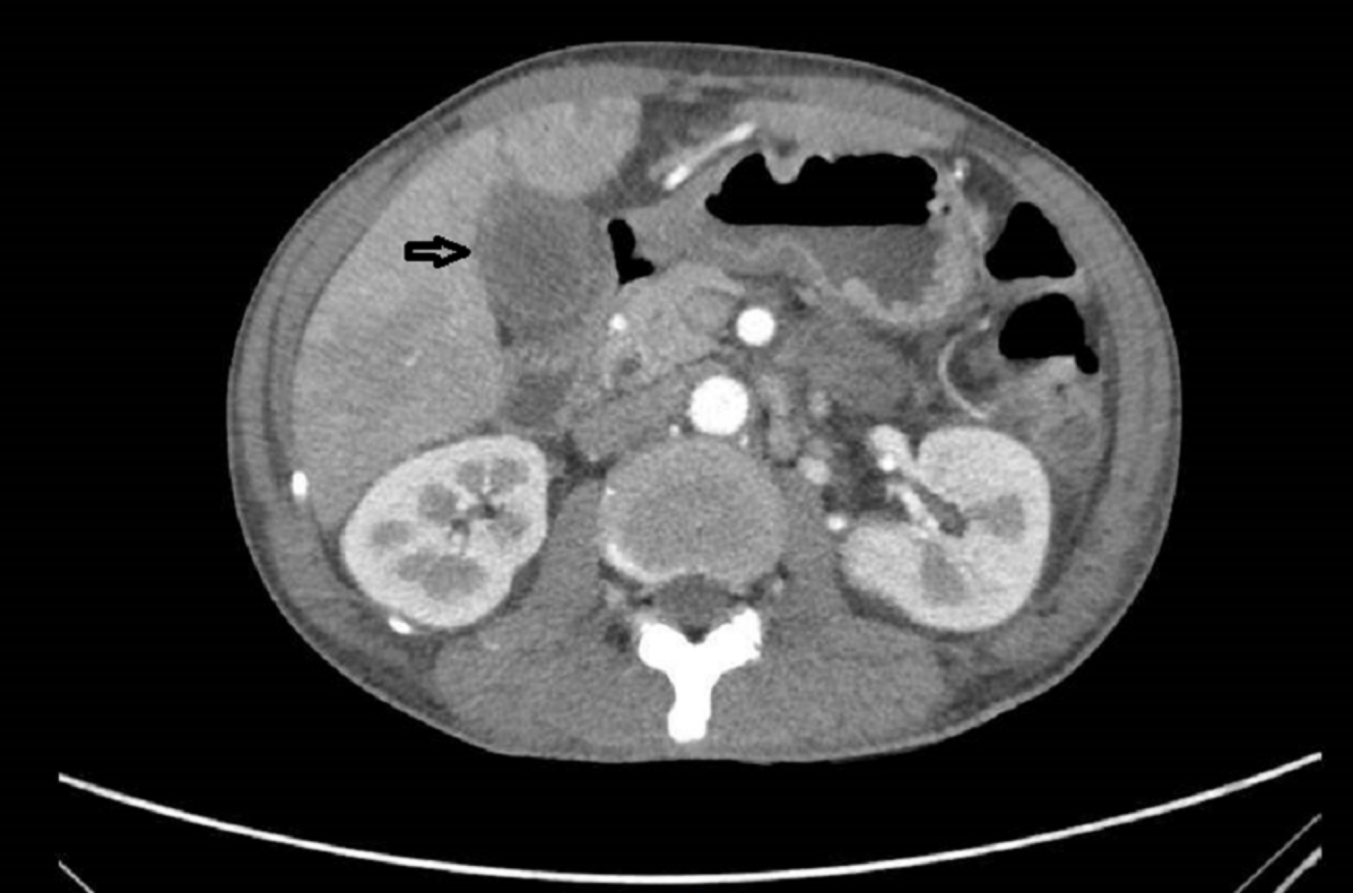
Computed tomography scan on admission The arrow indicates the location of the oedematous gallbladder.
